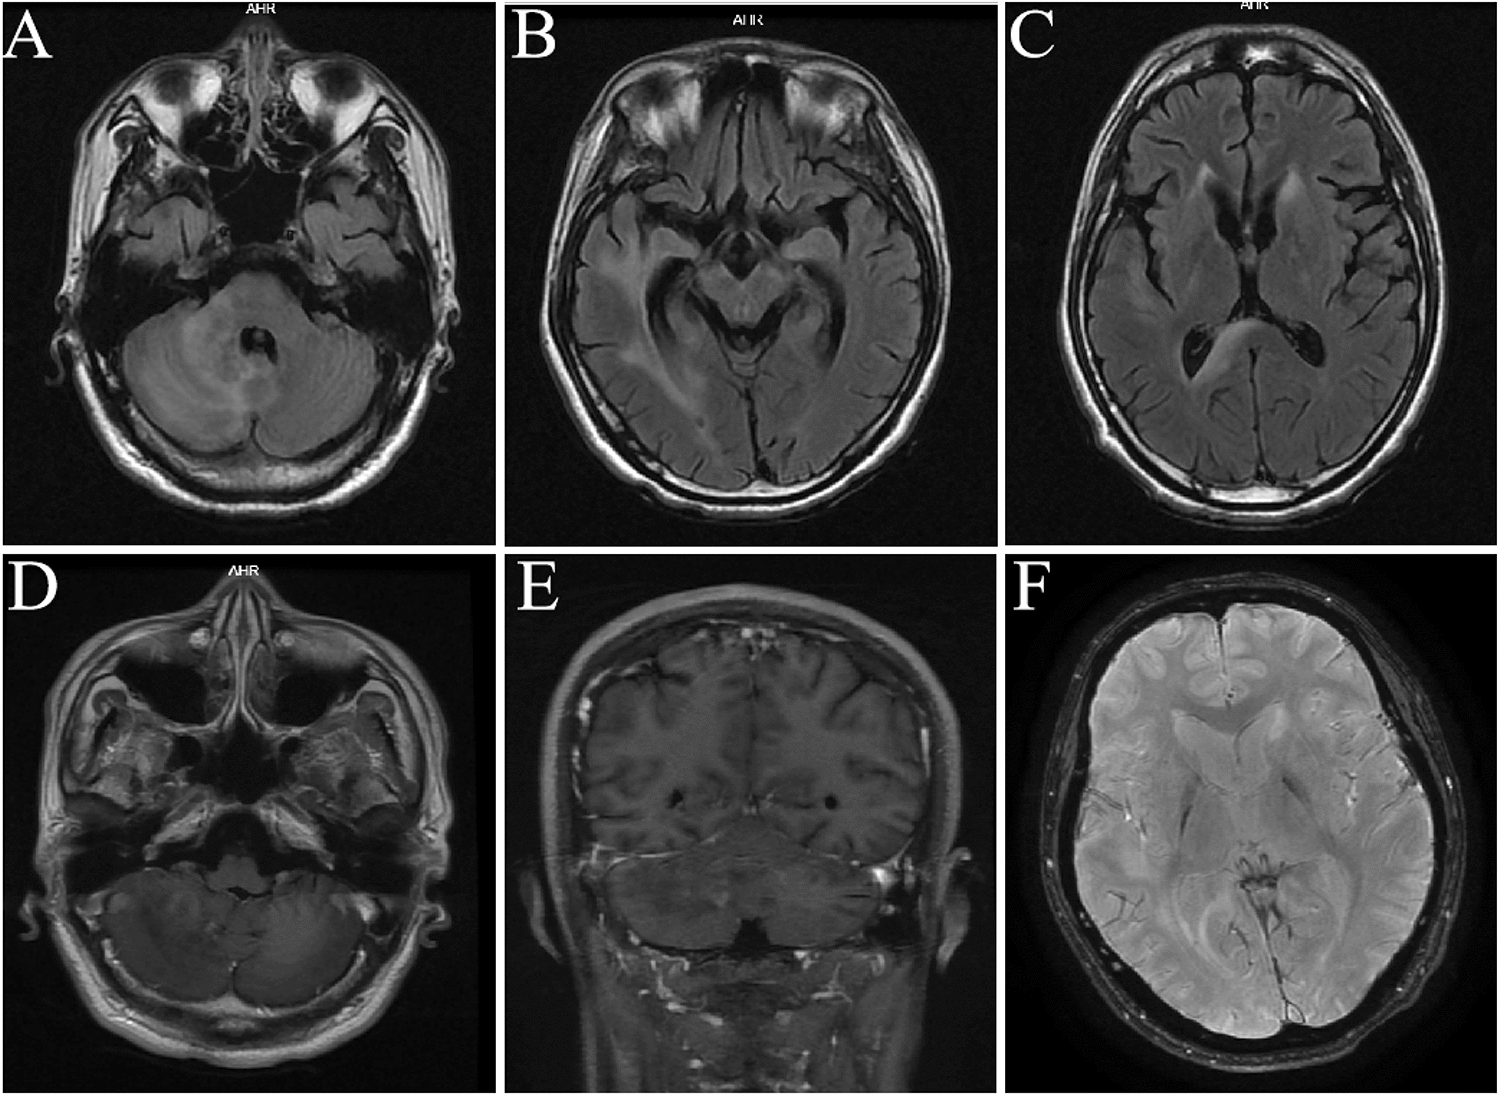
Axial T2-weighted FLAIR head MRI shows hyper-intense lesions in the right cerebellar hemisphere, brain stem, temporal lobe, and corpus callosum (A–C). Axial T1-weighted image show lesions in the right cerebellum were punctate enhancing after gadolinium administration (D, E). SWI scan was normal (F)
